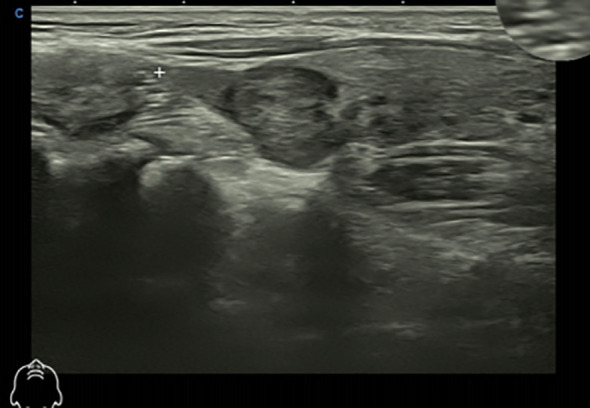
The patient’s color ultrasound showed hypoechoic nodules in the upper pole of the left lobe of the thyroid gland.
